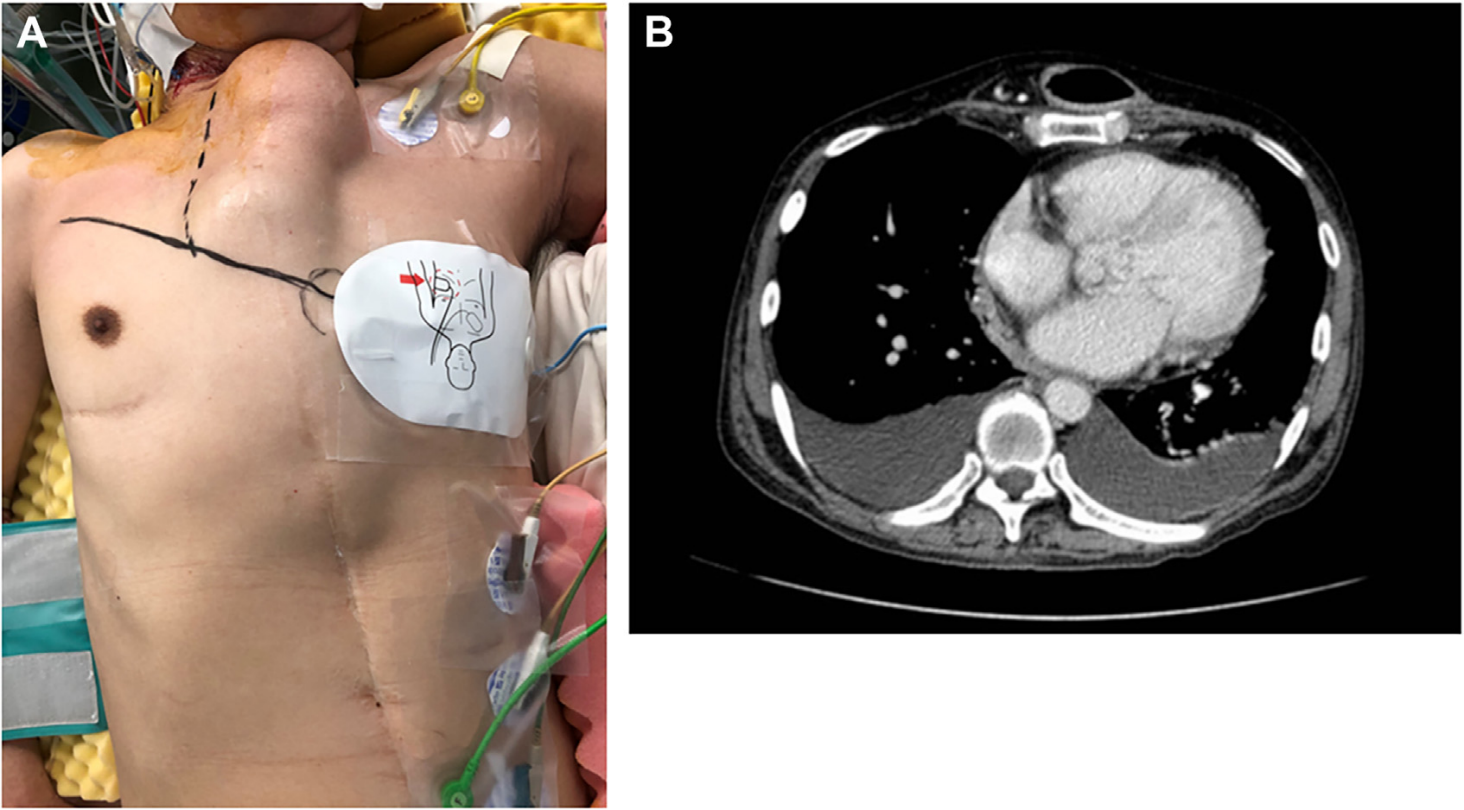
(A) A neoesophagus reconstructed through the anterosternal route is visible in the midline of the trunk. (B) Computed tomography shows that the neoesophagus is located on the sternum.
